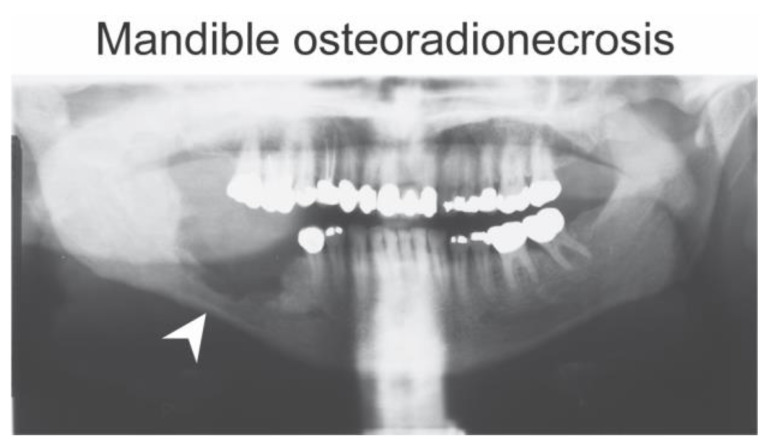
Mandible osteoradionecrosis. Panoramic radiograph of a head and neck cancer patient showing right mandible osteoradionecrosis (white arrowhead) that developed several months post-radiotherapy. The thin right mandibular cortical margin in the area of osteonecrosis poses a risk for potential pathological fracture of the mandible.
